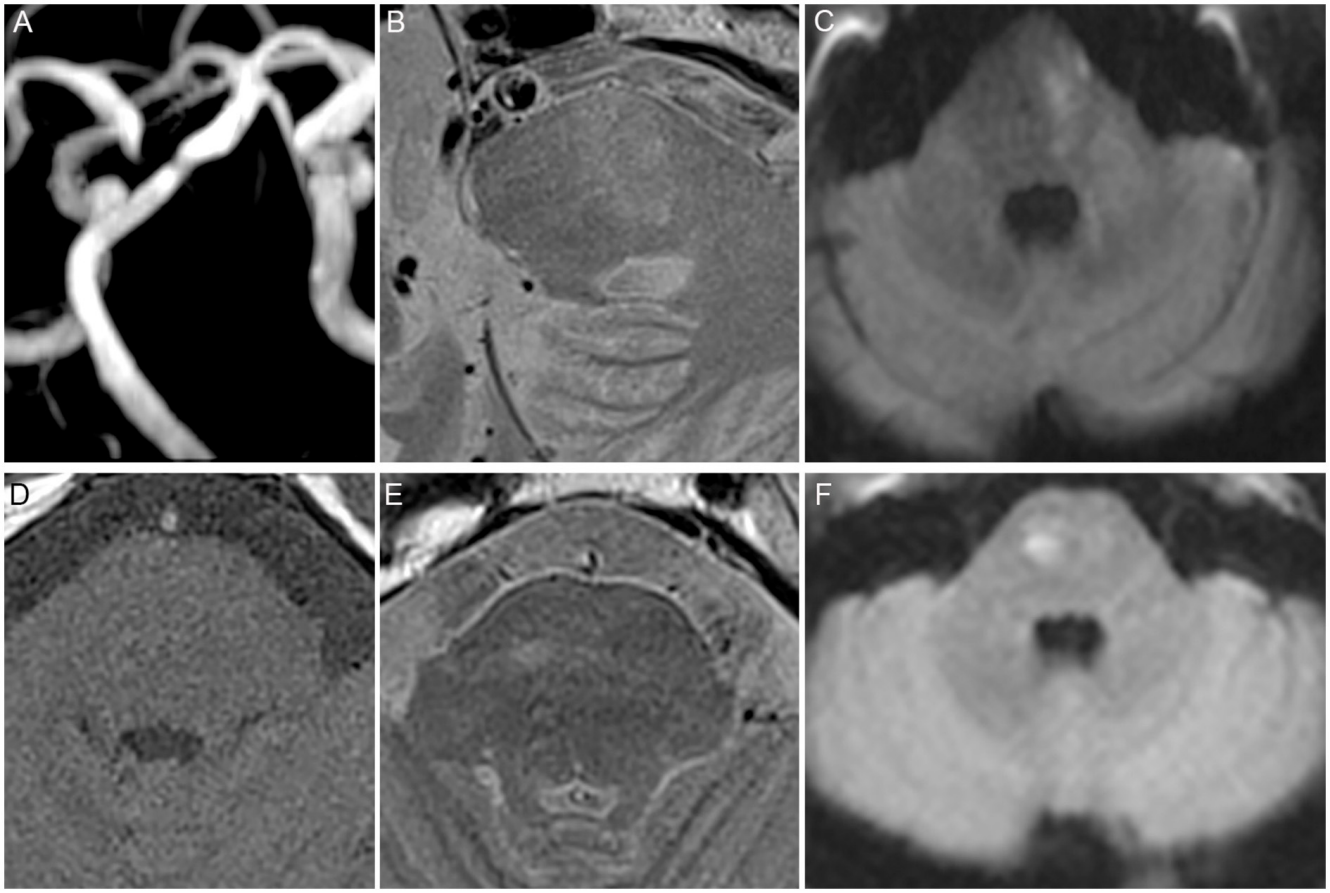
High risk features of the basilar artery (BA). (A) Significant BA stenosis. (B) Ruptured fibrous cap (RFC). (C) Acute infarction identified by diffusion-weighted imaging (DWI) located in the pons. (D) T1 and. (E) T2 showed abnormal signal. (F) Acute infarction identified by DWI located in the pons.
